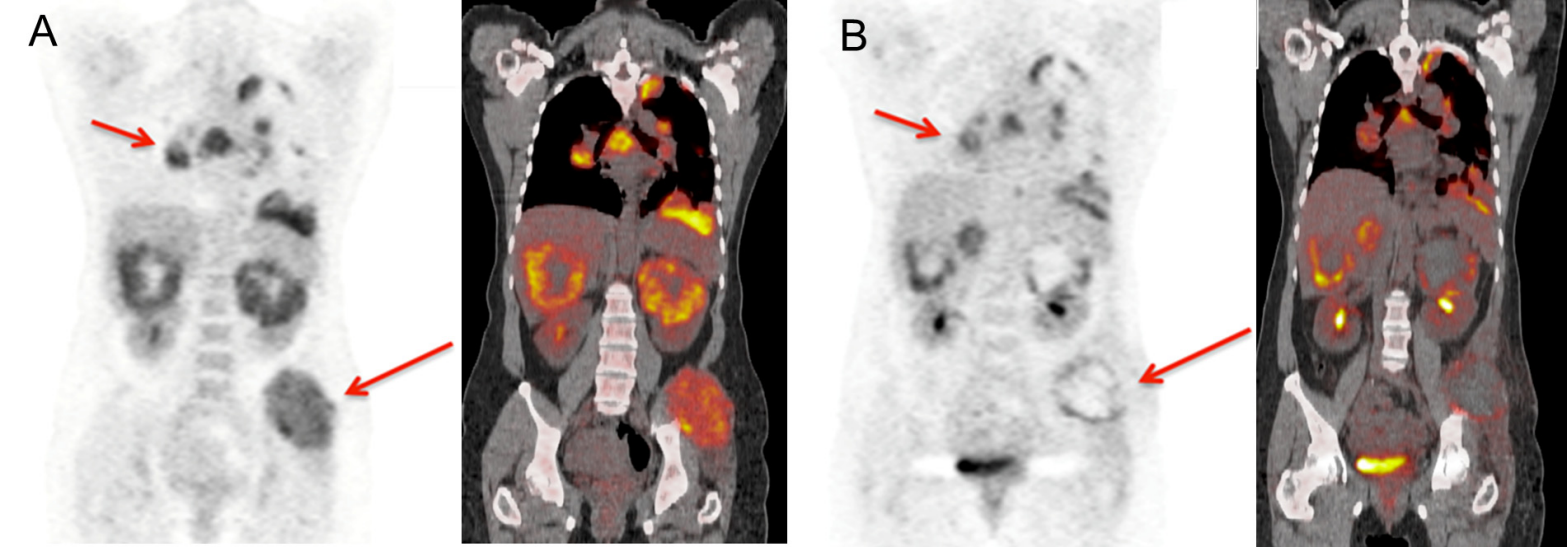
Baseline and post-cycle 2 FDG PET/CT demonstrates improved metabolic activity through multiple bulky foci at the interim therapy scan (red arrows). Size measurements on post-cycle 2 MRI (not shown) had also depicted overall lesion shrinkage. However, patient experienced early progression at 6 months.
